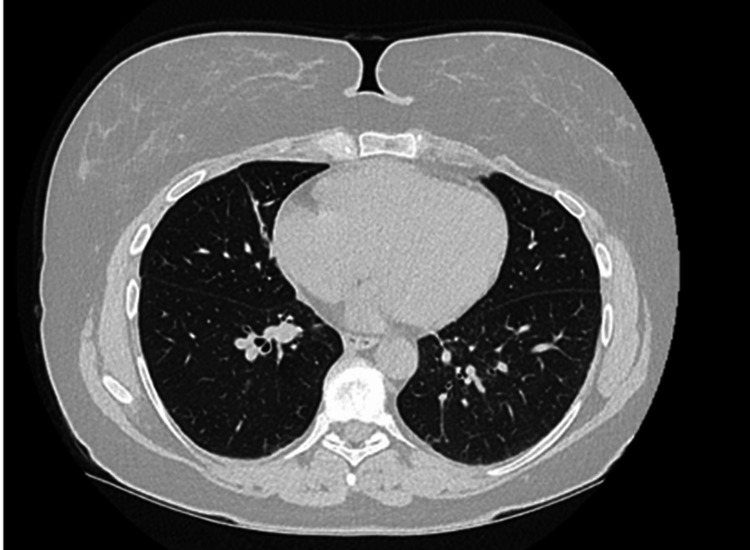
CT thorax with contrast after discontinuation of vedolizumab showing resolution of interstitial changes.
